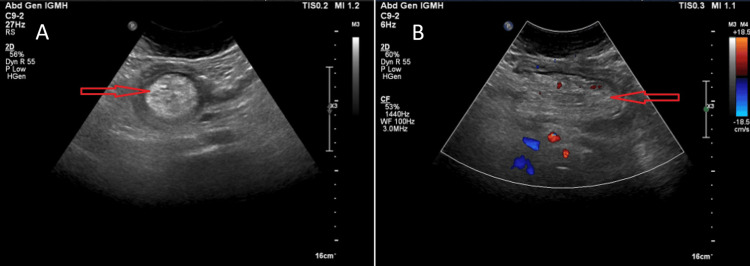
Abdominal ultrasound showing the intussusception (A) Target sign (red arrow); (B) Pseudokidney sign (red arrow)
